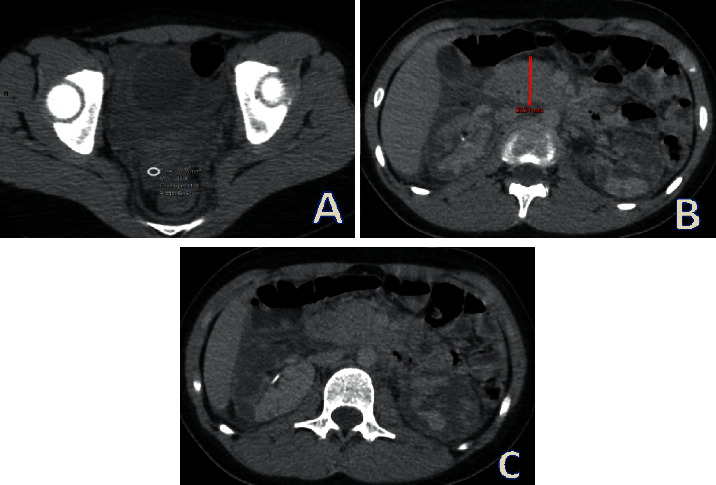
Abdomino-pelvic CT scan performed 12 hours after trauma, axial view. (a) Liquid of similar density as blood: haemoperitoneum. (b) Increase of pancreatic head size: pancreatitis. (c) Heterogeneous renal parenchyma with decreased enhancement during venous phase indicating ischemic lesions and necrosis.
